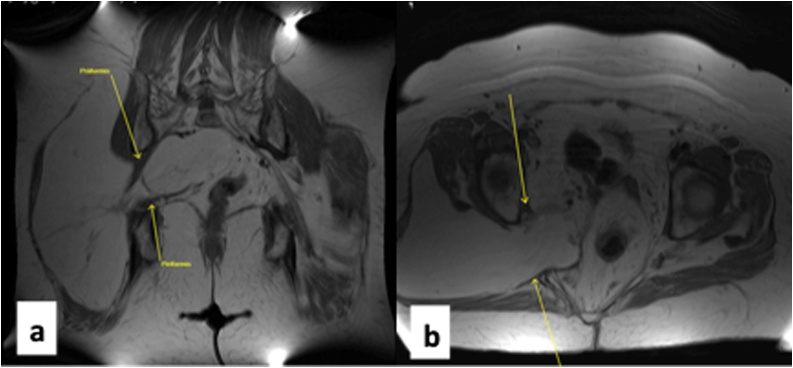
(a) MRI sagittal image of pelvis showing the tumor herniating through the sciatic foramen. (b) MRI axial image showing leftward displacement of the rectum.
